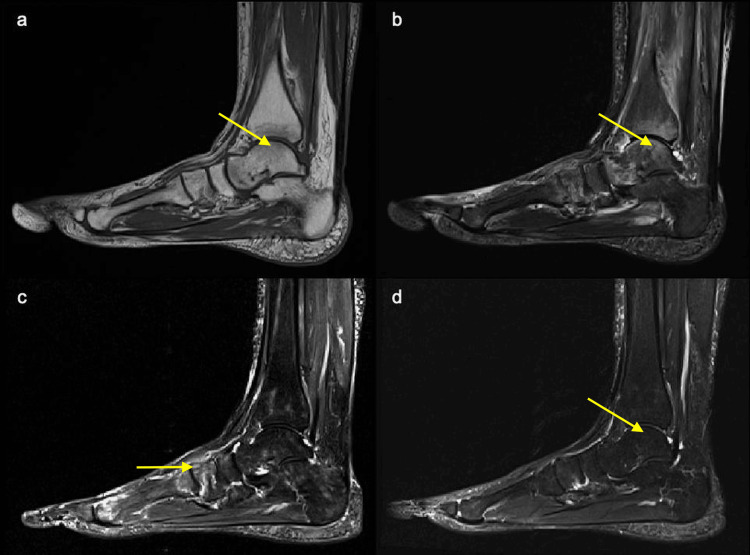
Magnetic resonance imaging (MRI) of the right ankle and foot (sagittal views). (a) T1-weighted image at one week postpartum showing low signal intensity in the distal tibia and tarsal bones. Arrow indicates a representative area in the talus. (b) Short tau inversion recovery (STIR) image at one week postpartum showing corresponding high signal intensity, indicating extensive bone marrow edema in the distal tibia, talus, calcaneus, cuboid, medial cuneiform, and proximal metatarsal bones. Arrow indicates bone marrow edema in the talus. (c) STIR image at three months postpartum showing the migration of bone marrow edema toward the forefoot. Arrow indicates bone marrow edema in the forefoot. (d) STIR image at eight months postpartum demonstrating the resolution of bone marrow edema in the ankle and foot. Arrow indicates the previously affected area in the talus.
